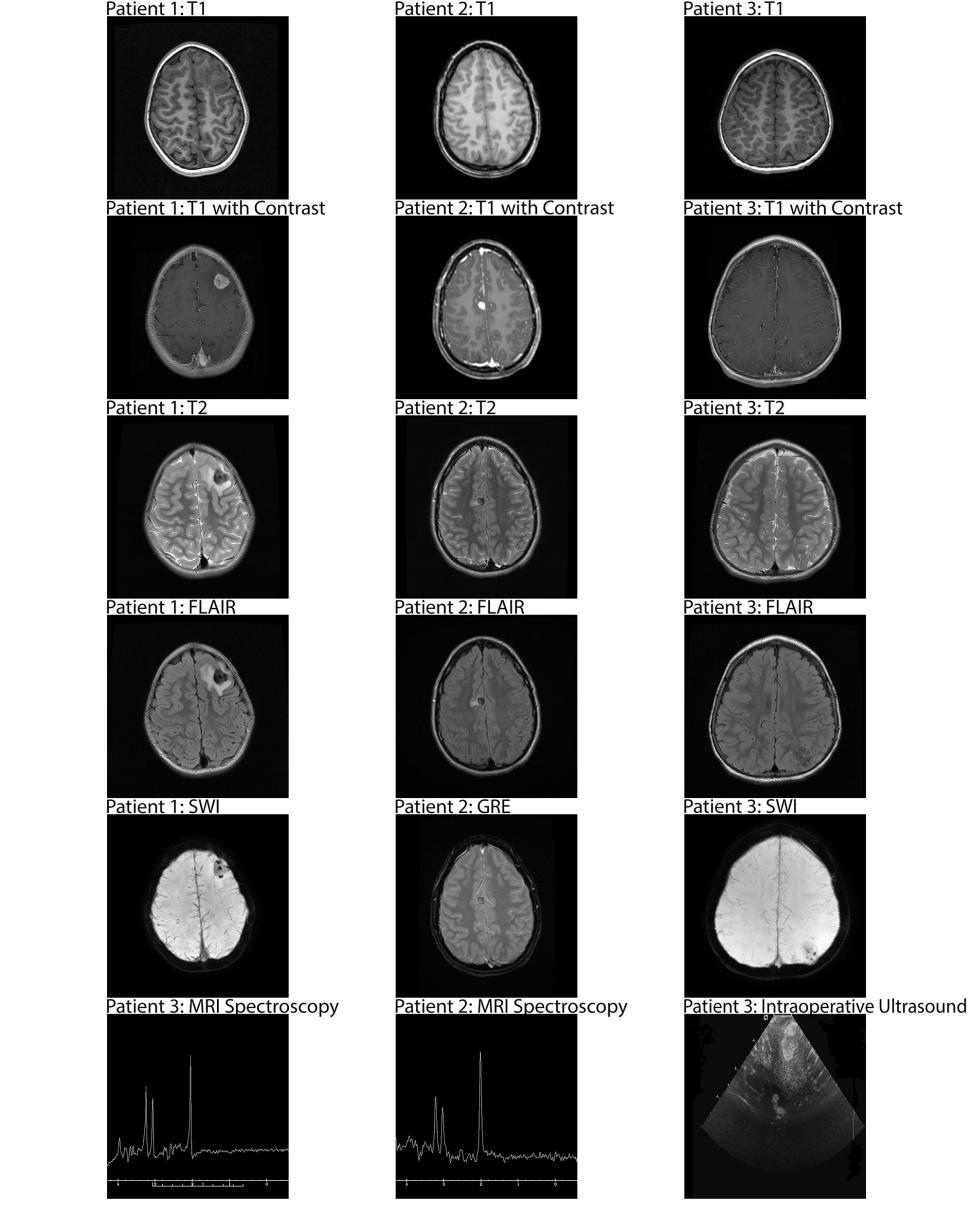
MRI brain, MRI spectroscopy, and intraoperative ultrasound of three patients treated in our center for MA. MA: Meningioangiomatosis; MRI: Magnetic resonance imaging.
